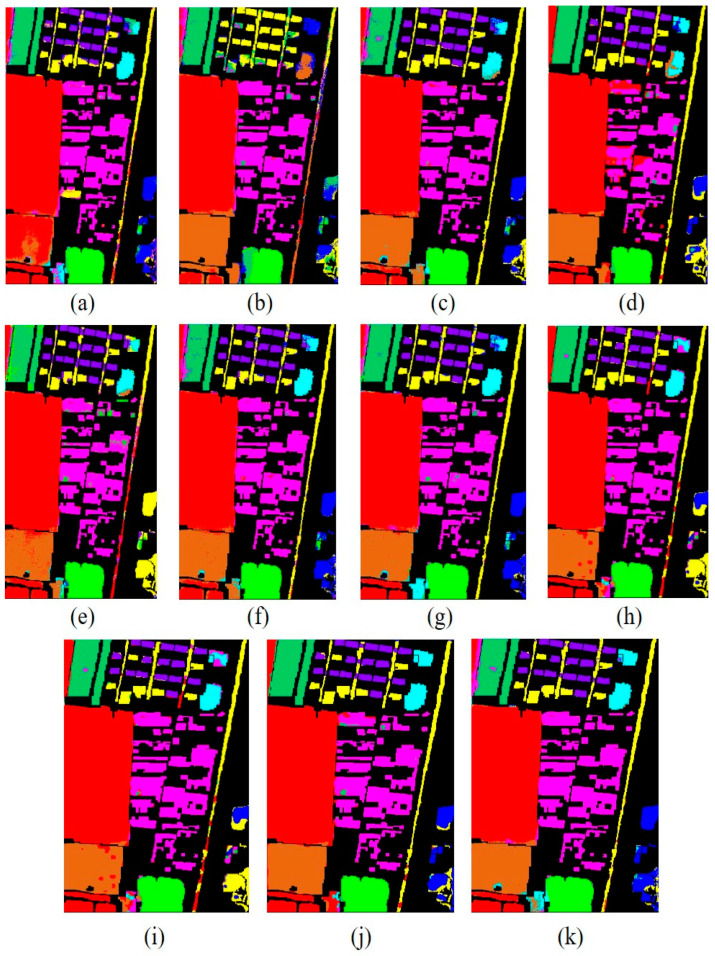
Classification maps obtained by different methods for the Xuzhou dataset. (a) SVM, (b) 2D, (c) 3D, (d) Hybrid, (e) DFFN, (f) Bam-CM, (g) ViT, (h) ST, (i) CT Mixer, (j) SSFTT, and (k) proposed.
